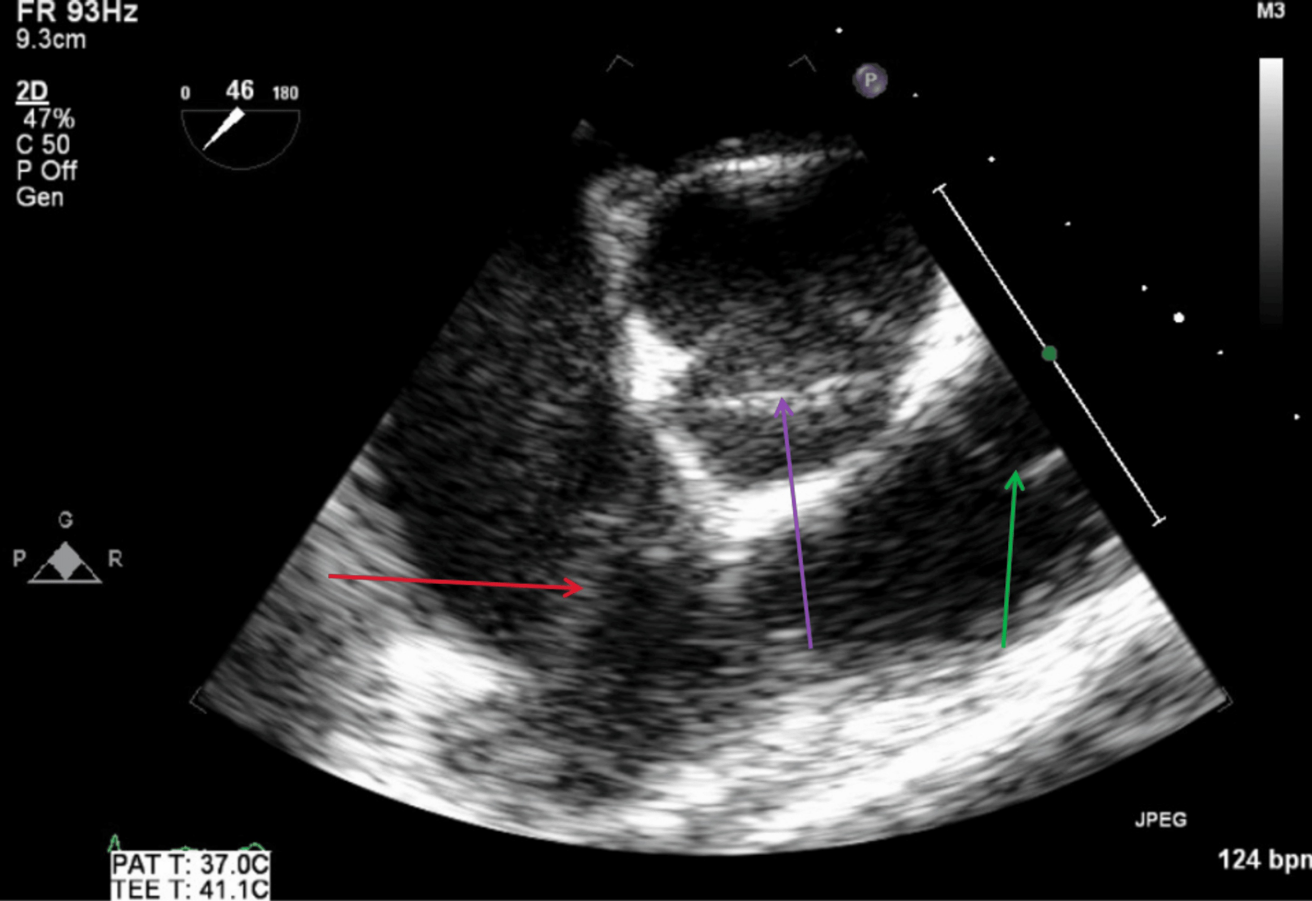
TEE mid-esophageal short-axis aortic valve view during diastole. The red arrow shows tricuspid valve with no visible vegetations. The purple arrow shows aortic valve with no visible vegetations. The green arrow shows pulmonic valve with no visible vegetations. TEE: transesophageal echocardiography
